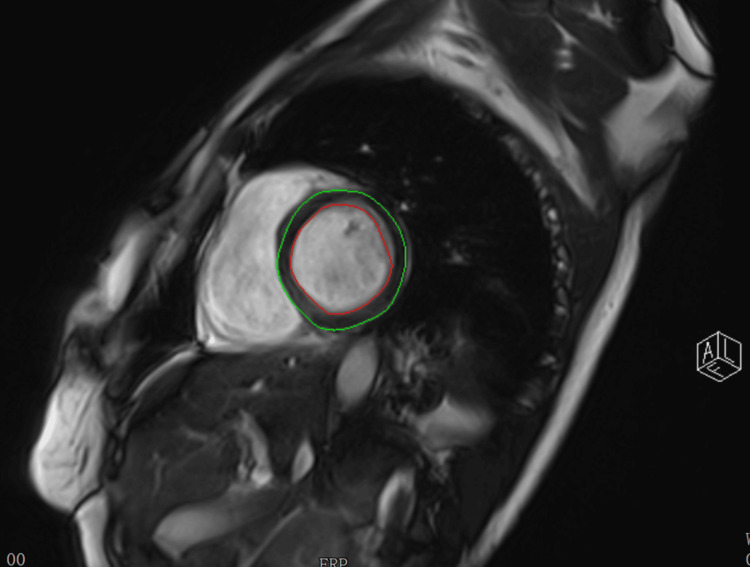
Cine segmented three-chamber cardiac magnetic resonance Image demonstrating a rounded nodular lesion (10 × 7 mm) arising from the posterior leaflet of the mitral valve, projecting toward the left atrium (circled). The lesion shows well-defined margins and imaging characteristics compatible with papillary fibroelastoma.
